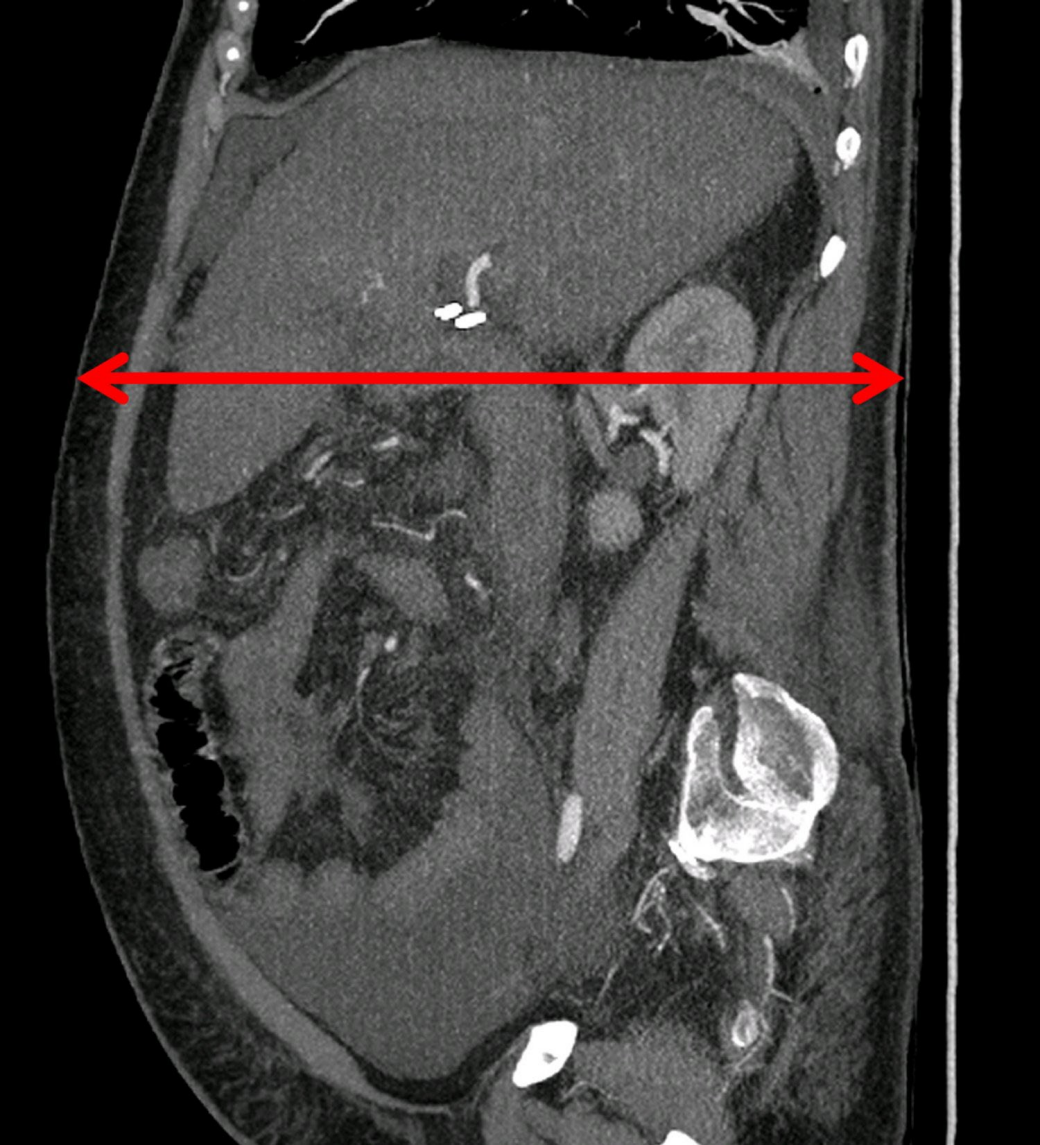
Image from postprocedural CT in Case 3 that was used to measure patient thickness in the anteroposterior direction. Patient thickness was measured at the location of the newly created shunt, which is visible in the image.
